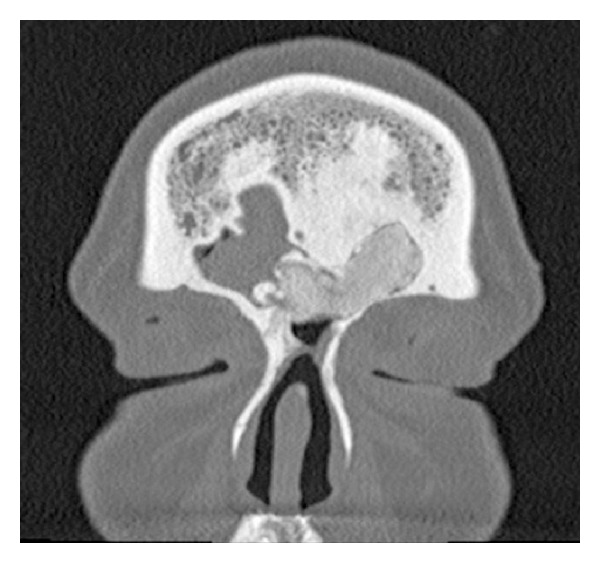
Coronal CT scan showing the osseous mass filling the left frontal sinus and extending into the right.
